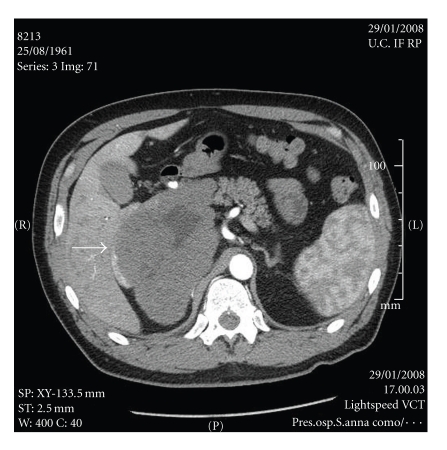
Pre-operative CT scan showing the huge hepatic mass arising from the caudate lobe with a right sided dislocation of the inferior vena cava (arrow).
